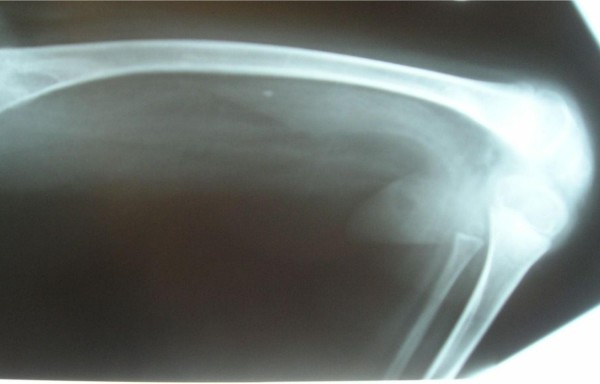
Anteroposterior radiograph of the lower limb showed significant fixed flexion deformity of 95° associated with minimal sclerosis around the metaphysis.
